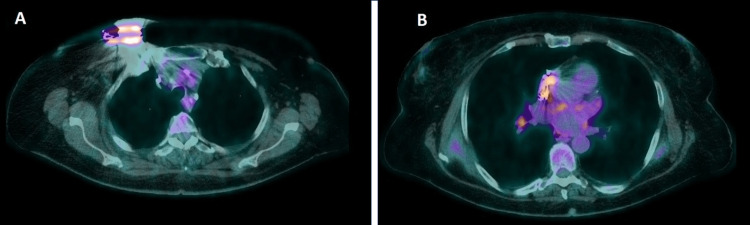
Transaxial views of fused 18F-FDG PET/CT images revealing signs suggestive of endocarditis due to an infected pacemaker. Findings (A and B) strongly support the diagnosis of CDRIE in the current clinical context. 18F-FDG PET/CT: Fluorine 18-fluorodeoxyglucose positron emission tomography/computed tomography. CDRIE:  Culture-negative endocarditis due to the infected pacemaker.
